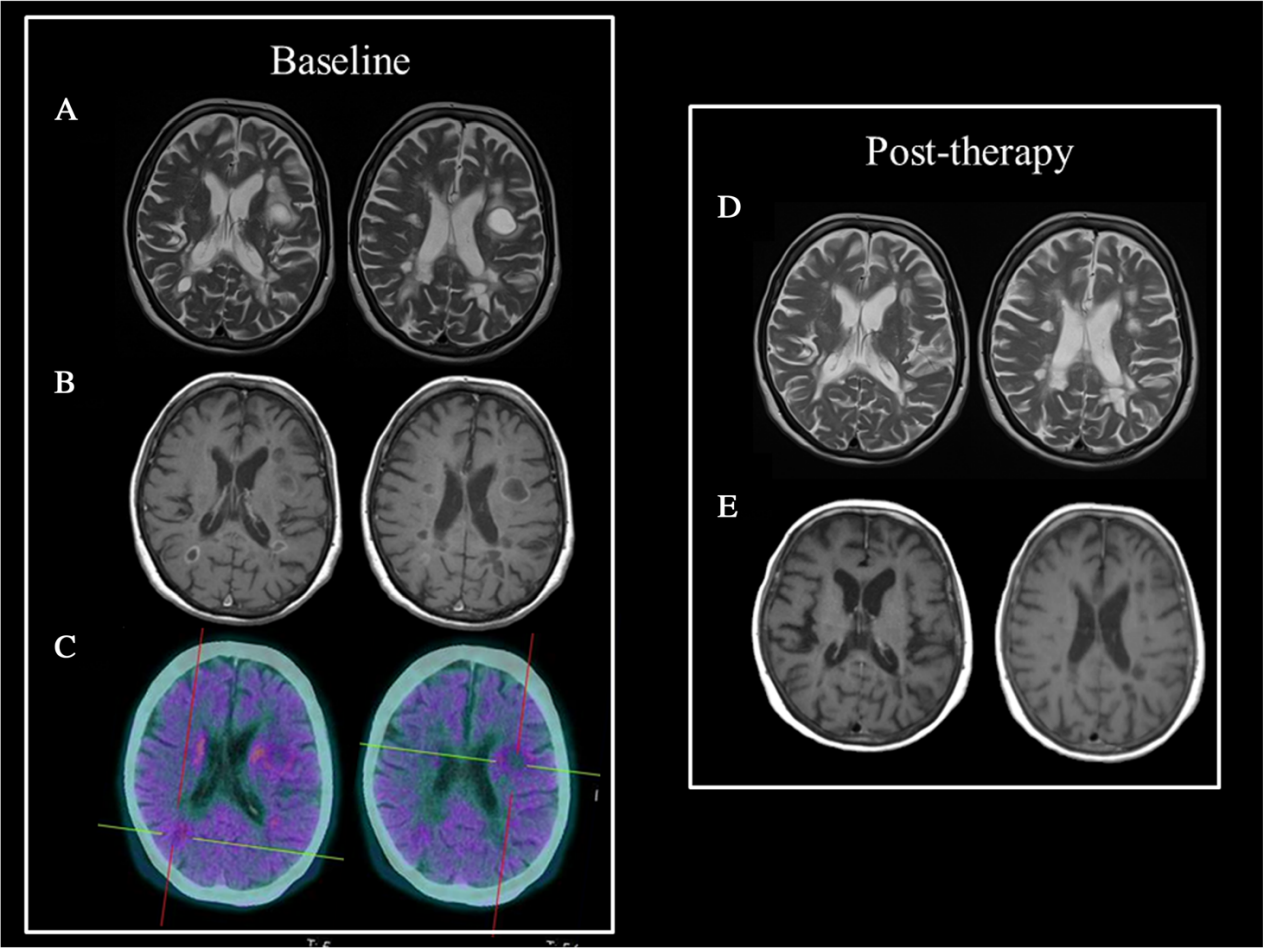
AA-PET and MRI findings in a case of differential diagnosis between TML and breast cancer brain metastasis. 71 years old female patient previously treated for breast cancer who underwent ceMRI that showed the presence of a left frontal lesion hyperintense at T2 sequences (Panel a) and hypointense at T1 sequences (Panel b). AA-PET/CT with ^18^F-Dopa showed a low tracer uptake supporting the hypothesis of TML. ceMRI performed three months after therapy administration showed the reduction in both lesion dimension (Panel d) and enhancement (Panel e)
